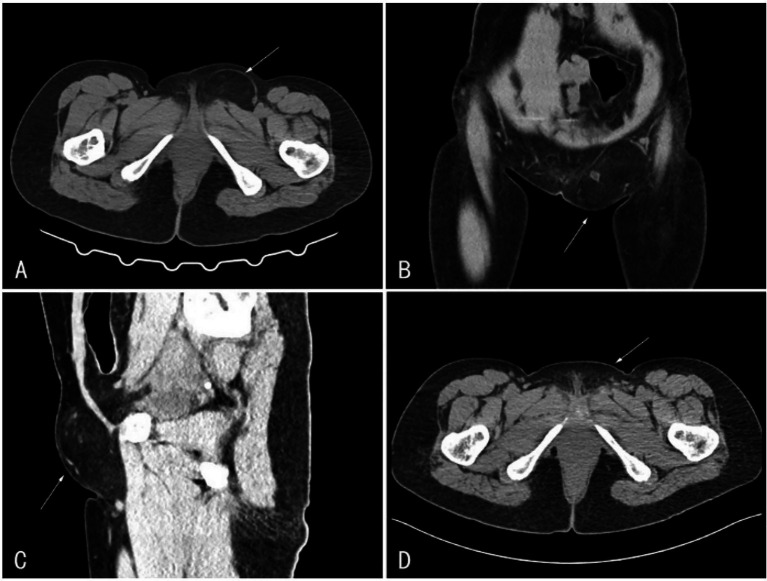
Perioperative CT imaging of the patient. (A) Preoperative coronal CT image of the inguinal region demonstrating a subcutaneous lipoma, indicated by the arrow. (B) Preoperative three-dimensional reconstructed CT image of the inguinal region showing the subcutaneous lipoma (arrow). (C) Preoperative lateral view of the three-dimensional reconstructed CT image of the inguinal region, with the subcutaneous lipoma indicated by the arrow. (D) Coronal CT image obtained 3 months postoperatively demonstrating complete resolution of the subcutaneous lipoma, with no evidence of seroma, hematoma, or lipoma recurrence.
